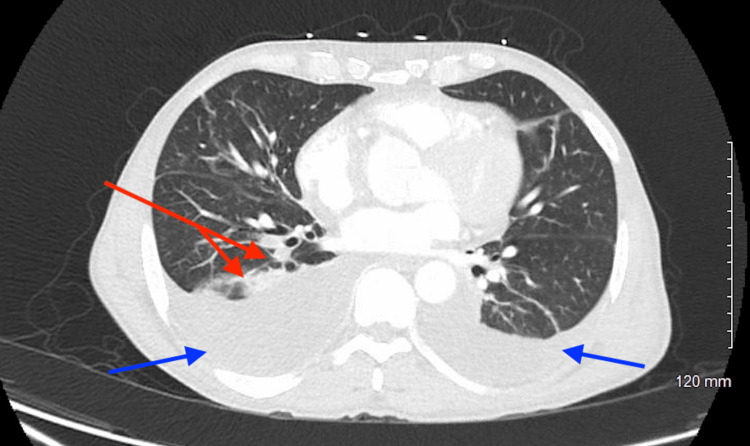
CT of the chest demonstrating right lower lobe peribronchiolar patchy opacities and bilateral pleural effusions. Red arrows = opacities; blue arrows = pleural effusions.
